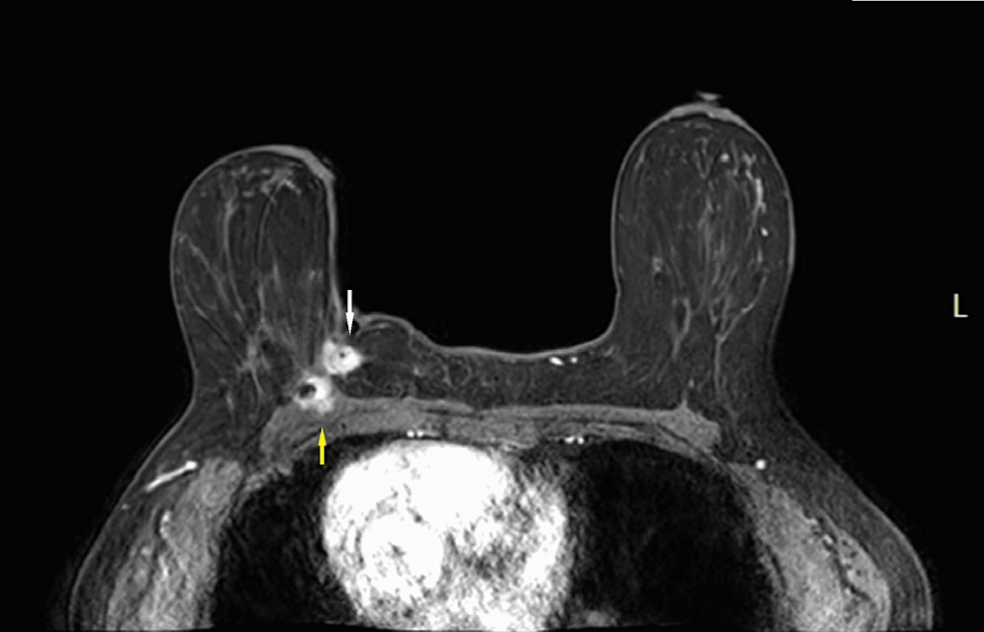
Post-contrast T1 fat-saturated MRI axial image at the time of presentation. Corresponding to the mammographic and ultrasound findings at the site of the surgical scar, there is an enhancing mass in the central inner right breast at posterior depth (white arrow) with pectoralis muscle invasion (yellow arrow).
